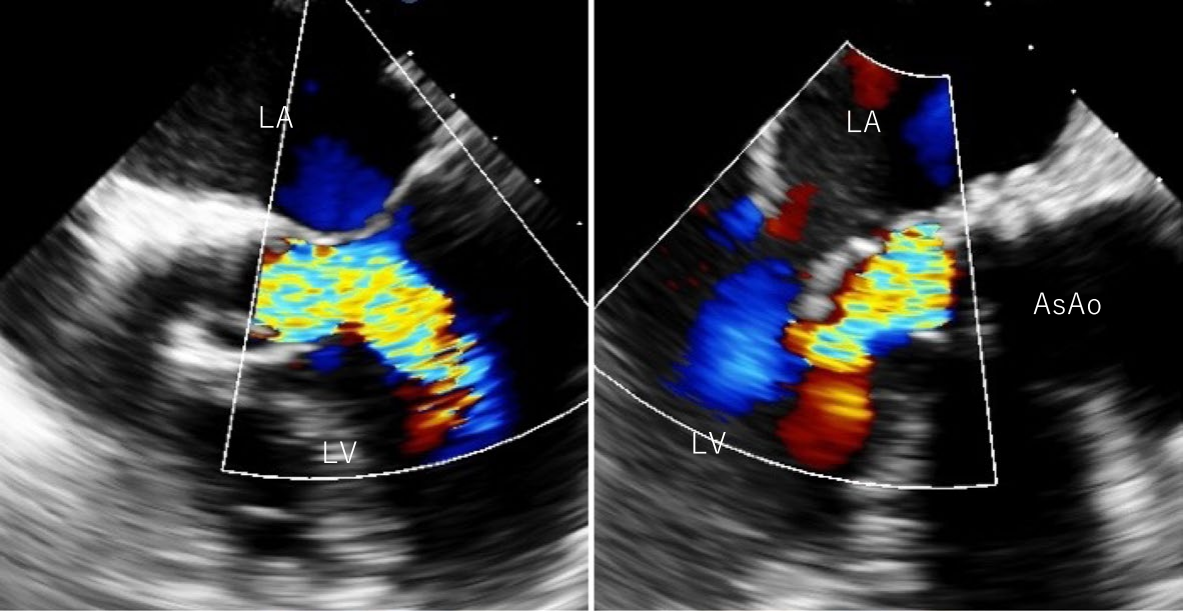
Intraoperative transesophageal echocardiographic view of case 2
